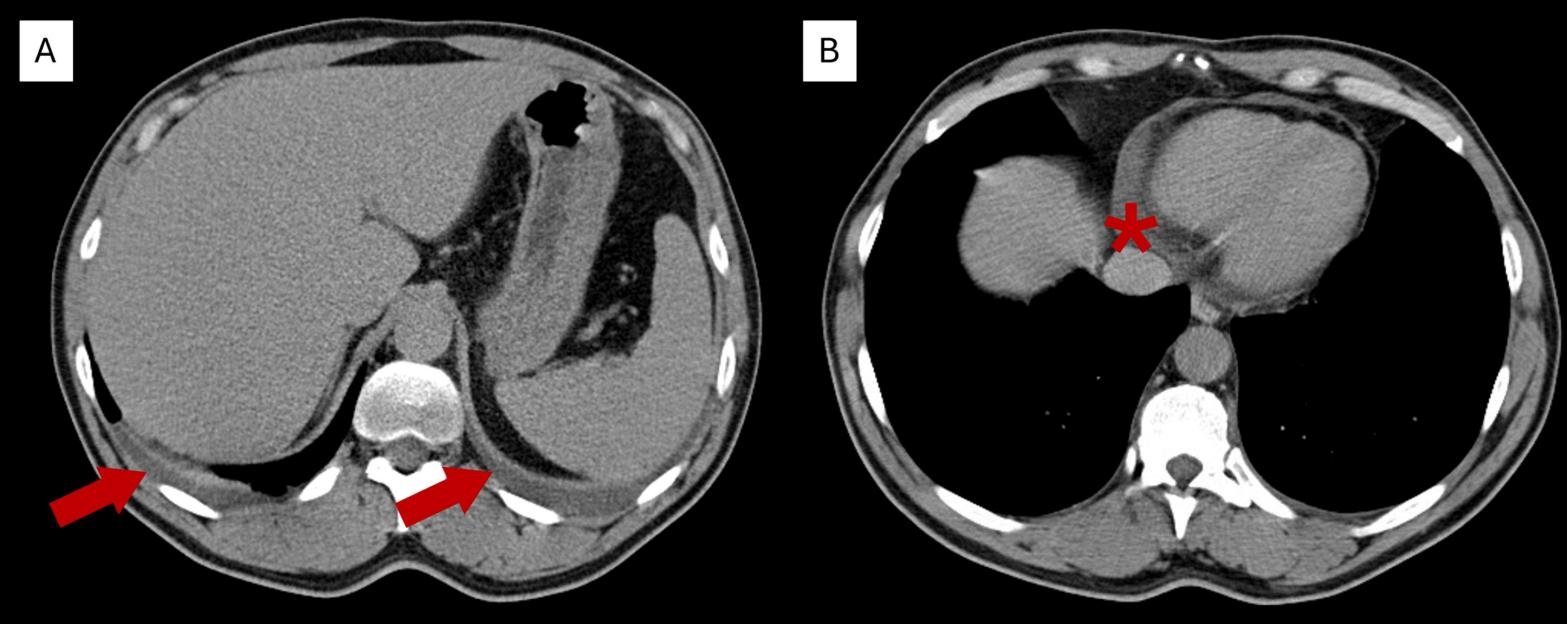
Axial contrast-enhanced CT scan. CT images showing (A) small bilateral pleural effusions (red arrows) and (B) a small pericardial effusion (*), consistent with mild serosal inflammation.
